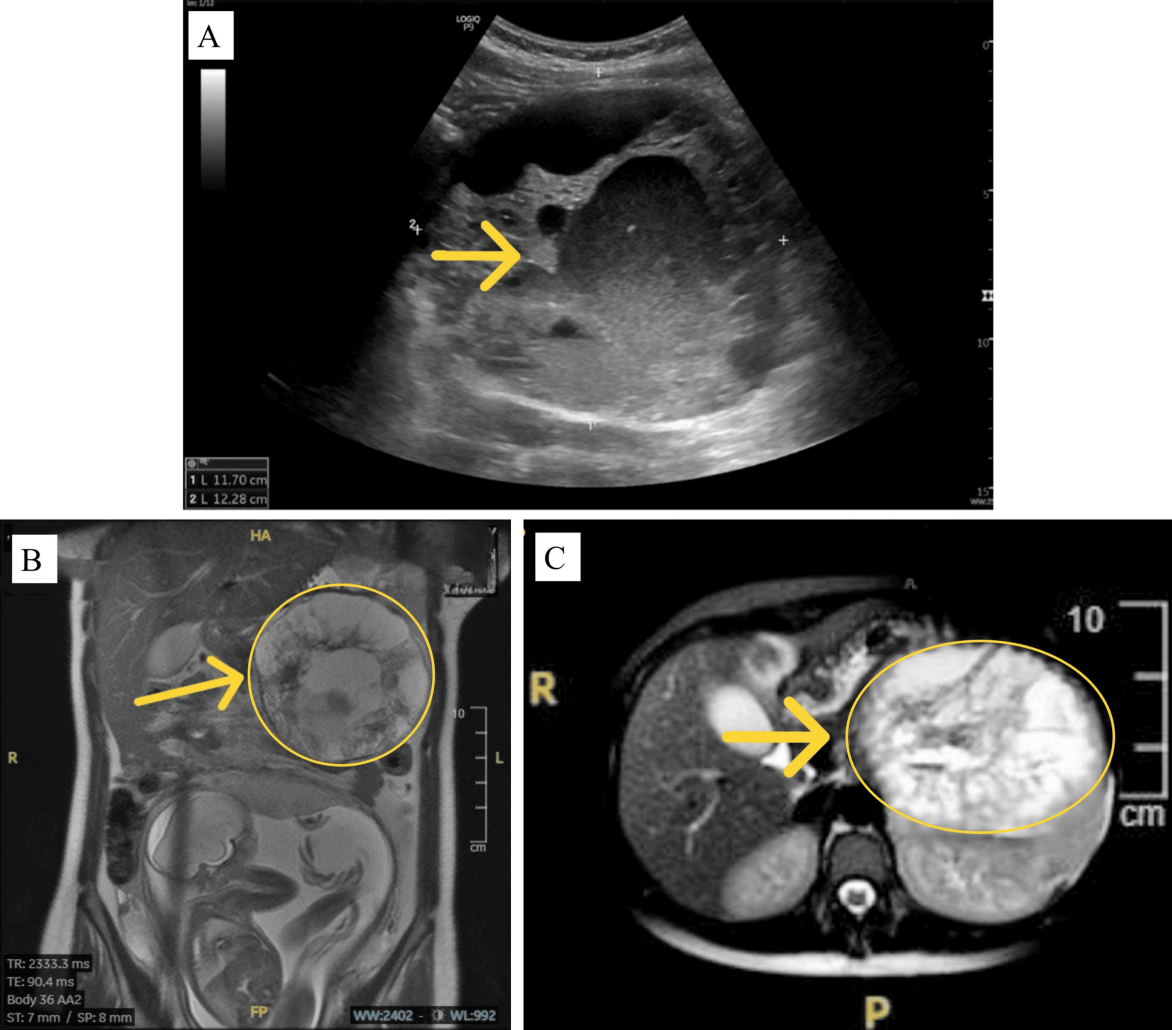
Ultrasound and MRI images of the abdomen before surgery. A: Abdominal ultrasound showing a large heterogeneous epigastric mass with cystic components (yellow arrow). B: Coronal T2-weighted MRI of the abdomen showing a large heterogeneous mass (yellow arrow) located anterior to the left kidney. The mass demonstrates mixed solid and cystic components, internal fluid levels, and peripheral hyperintense areas consistent with hemorrhagic or necrotic changes. It is displacing adjacent structures, including the pancreas and stomach. These imaging features were later confirmed to represent a solid pseudopapillary neoplasm (SPN) of pancreatic origin. C: Axial abdominal MRI scan demonstrating a large mixed solid-cystic tumor circled in yellow, displacing adjacent structures.
